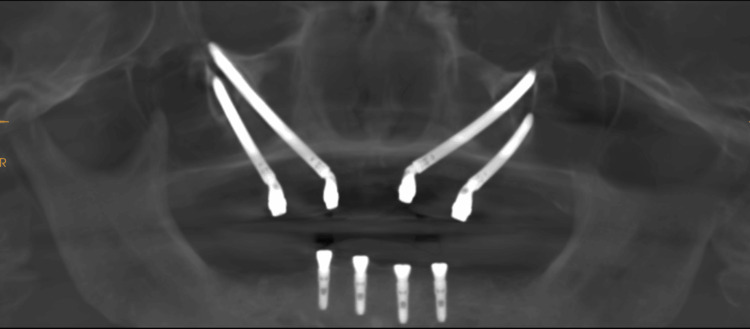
Post-operative CBCT panorama CBCT: Cone beam computed tomography
